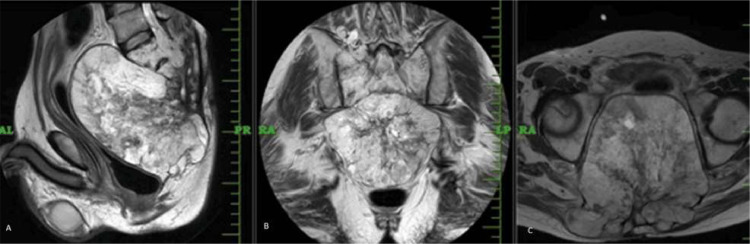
MRI T2 weighted sagittal (A), coronal (B), axial (C) sections showing heterogeneously hyperintense mass lesion in lower sacral and coccyx region.
